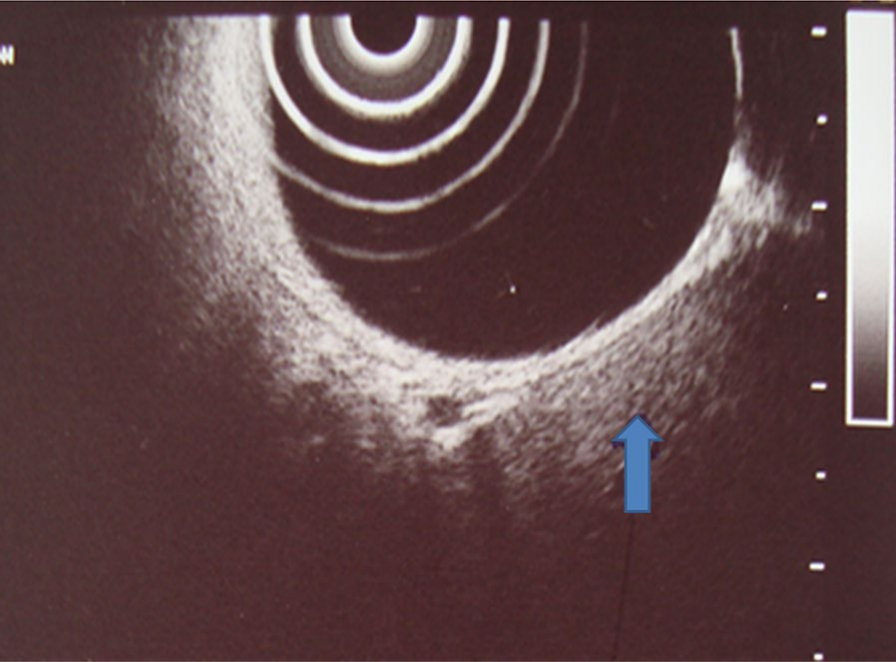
Upper GI endosonography showing a mass lesion in the fundus of stomach
